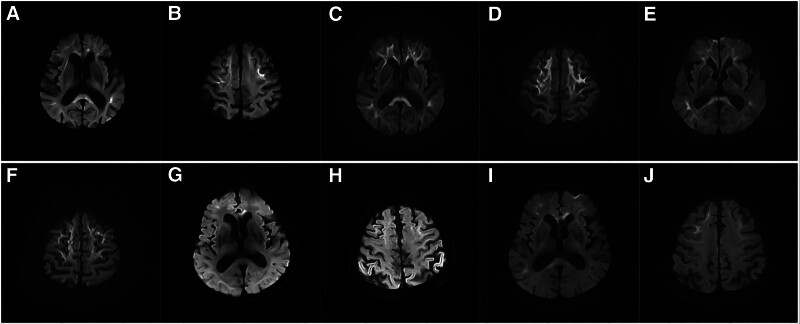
Brain MRI findings of the 3 patients. (A, B) show findings for Patient 1. Brain DWI in May 2019 shows hyperintensity in the corticomedullary junction of the bilateral frontoparietal, temporal, and occipital lobes and genu and splenium of the corpus callosum. (C, D) Brain DWI of the same patient in June 2023 shows hyperintensity in the corticomedullary junction of the bilateral frontoparietal, temporal, and occipital lobes and genu and splenium of the corpus callosum. Over the 4-year period from 2019 to 2023, the lesions had expanded to the posterior part of the brain, and the white matter lesions were slightly worse than those observed before. (E, F) show findings for Patient 2. Brain DWI in October 2023 shows hyperintensity in the corticomedullary junction of the bilateral frontoparietal, temporal, and occipital lobes and genu and splenium of the corpus callosum. (G–J) show findings for Patient 3 from 2020 to 2023; (G–H) represent findings in April 2020; (I–J) represent findings in May 2023. DWI hyperintensity was noted in the corticomedullary junction of the bilateral frontoparietal, temporal and occipital lobes and genu and splenium of the corpus callosum. The lesion expanded slightly to the posterior part of the brain over the 3-year period from 2020 to 2023. DWI = diffusion-weighted imaging, MRI = magnetic resonance imaging.
